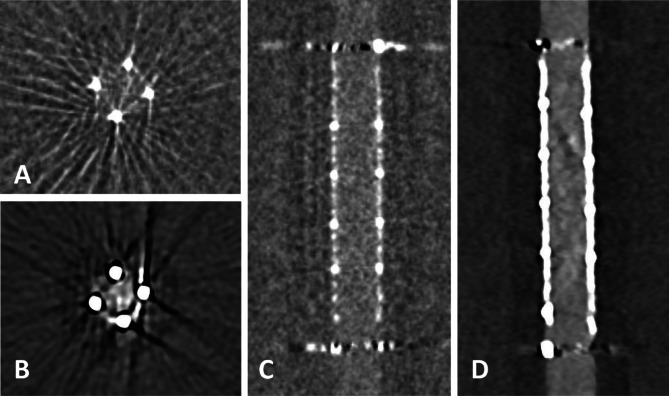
Flat panel CT (A, C) and photon-counting detector CT (CTDIvol 20 mGy; B, D) with axial and sagittal reconstructions of a FRED 4,5 × 20 × 13 mm flow diverter. Note the pronounced metal artifacts at the flow diverter ends and the limited assessability of the lumen in both modalities. Window settings were manually optimized for contrast: A, B) W: 700 HU, L: 2000 HU; C, D) W: 250 HU, L: 700 HU.
